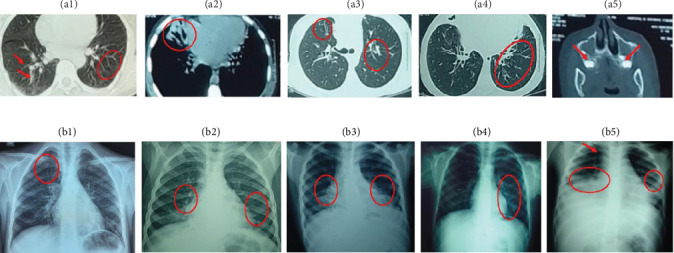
Radiographic and CT imaging of patients with MHC-II deficiency. (A) Chest CT scan images showing bronchiectasis in patient P1 (a1, a2) and patient P2 (a3, a4). Image a5 shows sinusitis in patient P2. (B) Chest X-ray images showing clarity on the upper left lobe with a mild distention in patient P1 (b1) and bronchiectasis associated with lobar condensation and mediastinal adenopathy in patient P2 (b2, b3, b4). Image b5 (P2) shows right lower lobe and left lower lobe condensation with significant thoracic distention, revealing pneumonia associated to mediastinal enlargement.
